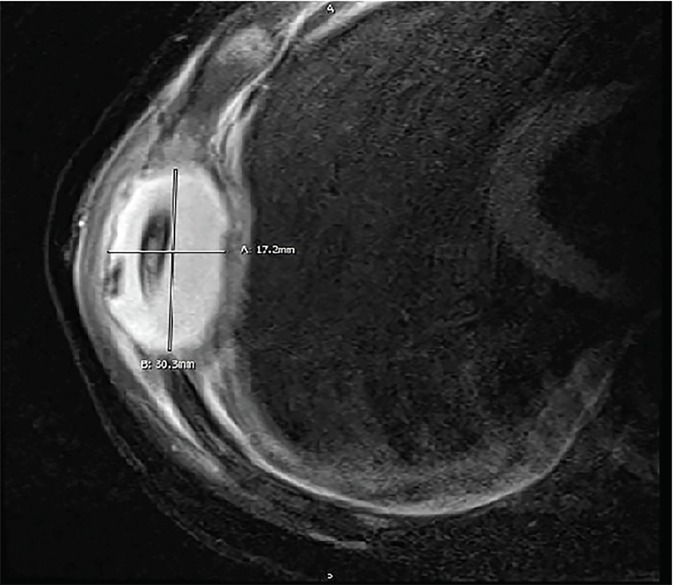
Magnetic resonance imaging (axial view: T1, T2 fat saturation technique) showing 17.2 × 30.3 mm periosteal abscess of rib.
